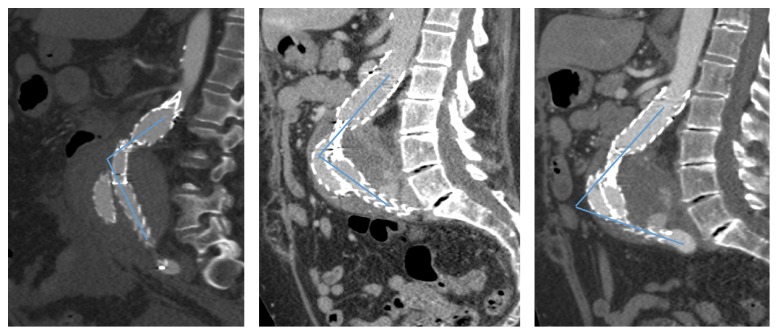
Sagittal view of computed tomography performed at 1, 6, and 12 months of follow-up demonstrating the anterior displacement of the aortic endograft.
